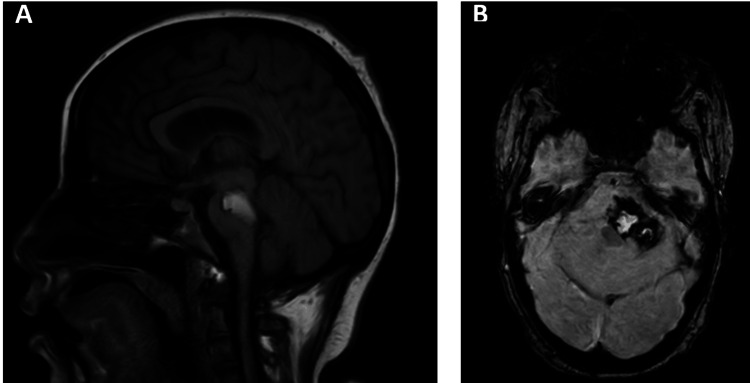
Saggital and axial images. (A) Sagittal T1-weighted MRI demonstrating a reduction in the extent of the hyperintense signal along the midbrain and pons (B) Axial T2*/SWI sequence showing a decrease in the size of the hyperintensity involving the pons, cerebral peduncle, and left cerebellar hemisphere.
